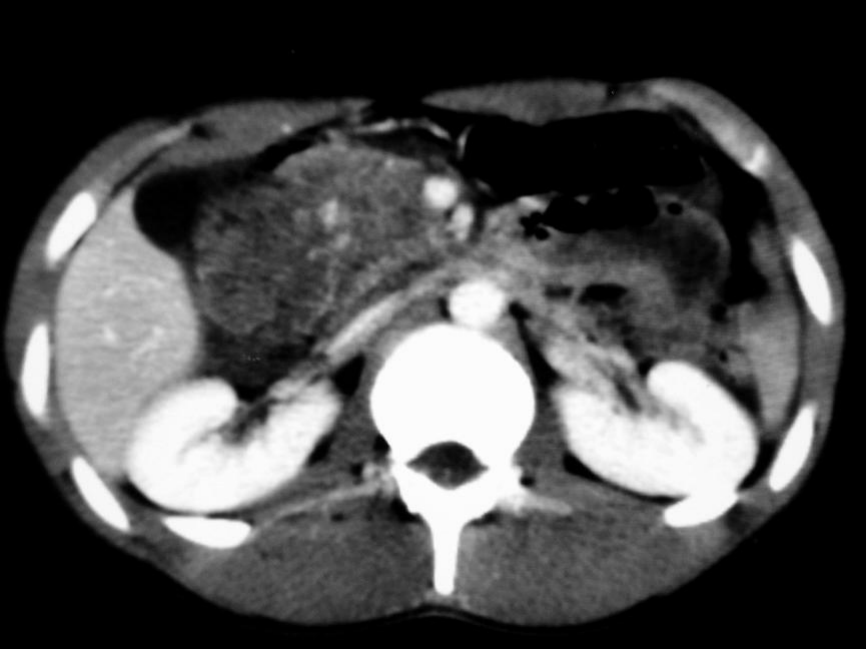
A computed tomography scan of the patient's abdomen showing a retroperitoneal haematoma at the duodenal level.
